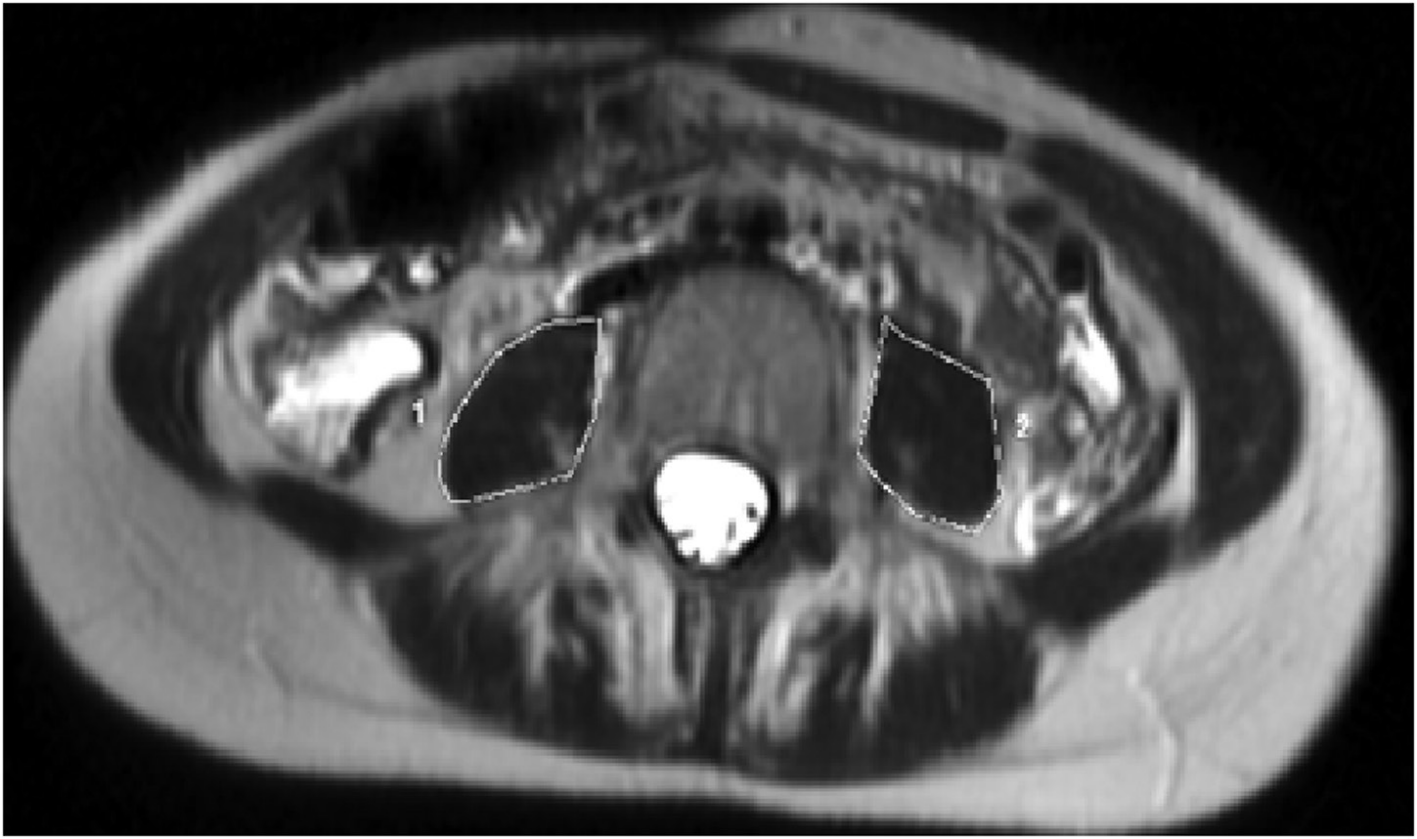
Demonstration of total psoas area calculation on an axial T2-weighted image.
